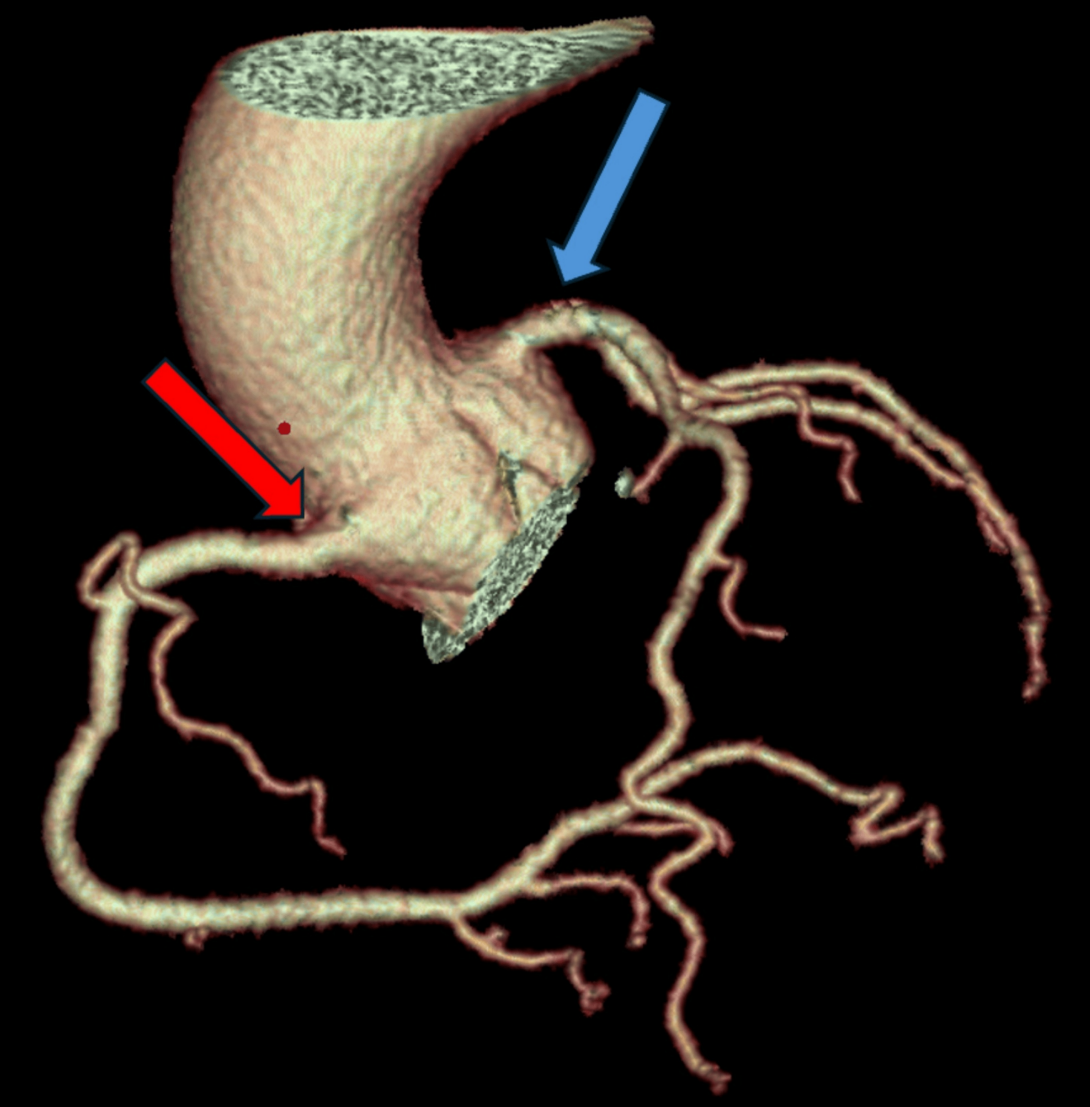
Computed tomography angiography with a 3D-rendered image demonstrating the normal course of the coronary arteries. The red arrow indicates the right coronary artery, originating from the right coronary cusp. The blue arrow indicates the left main artery, originating from the left coronary cusp.
